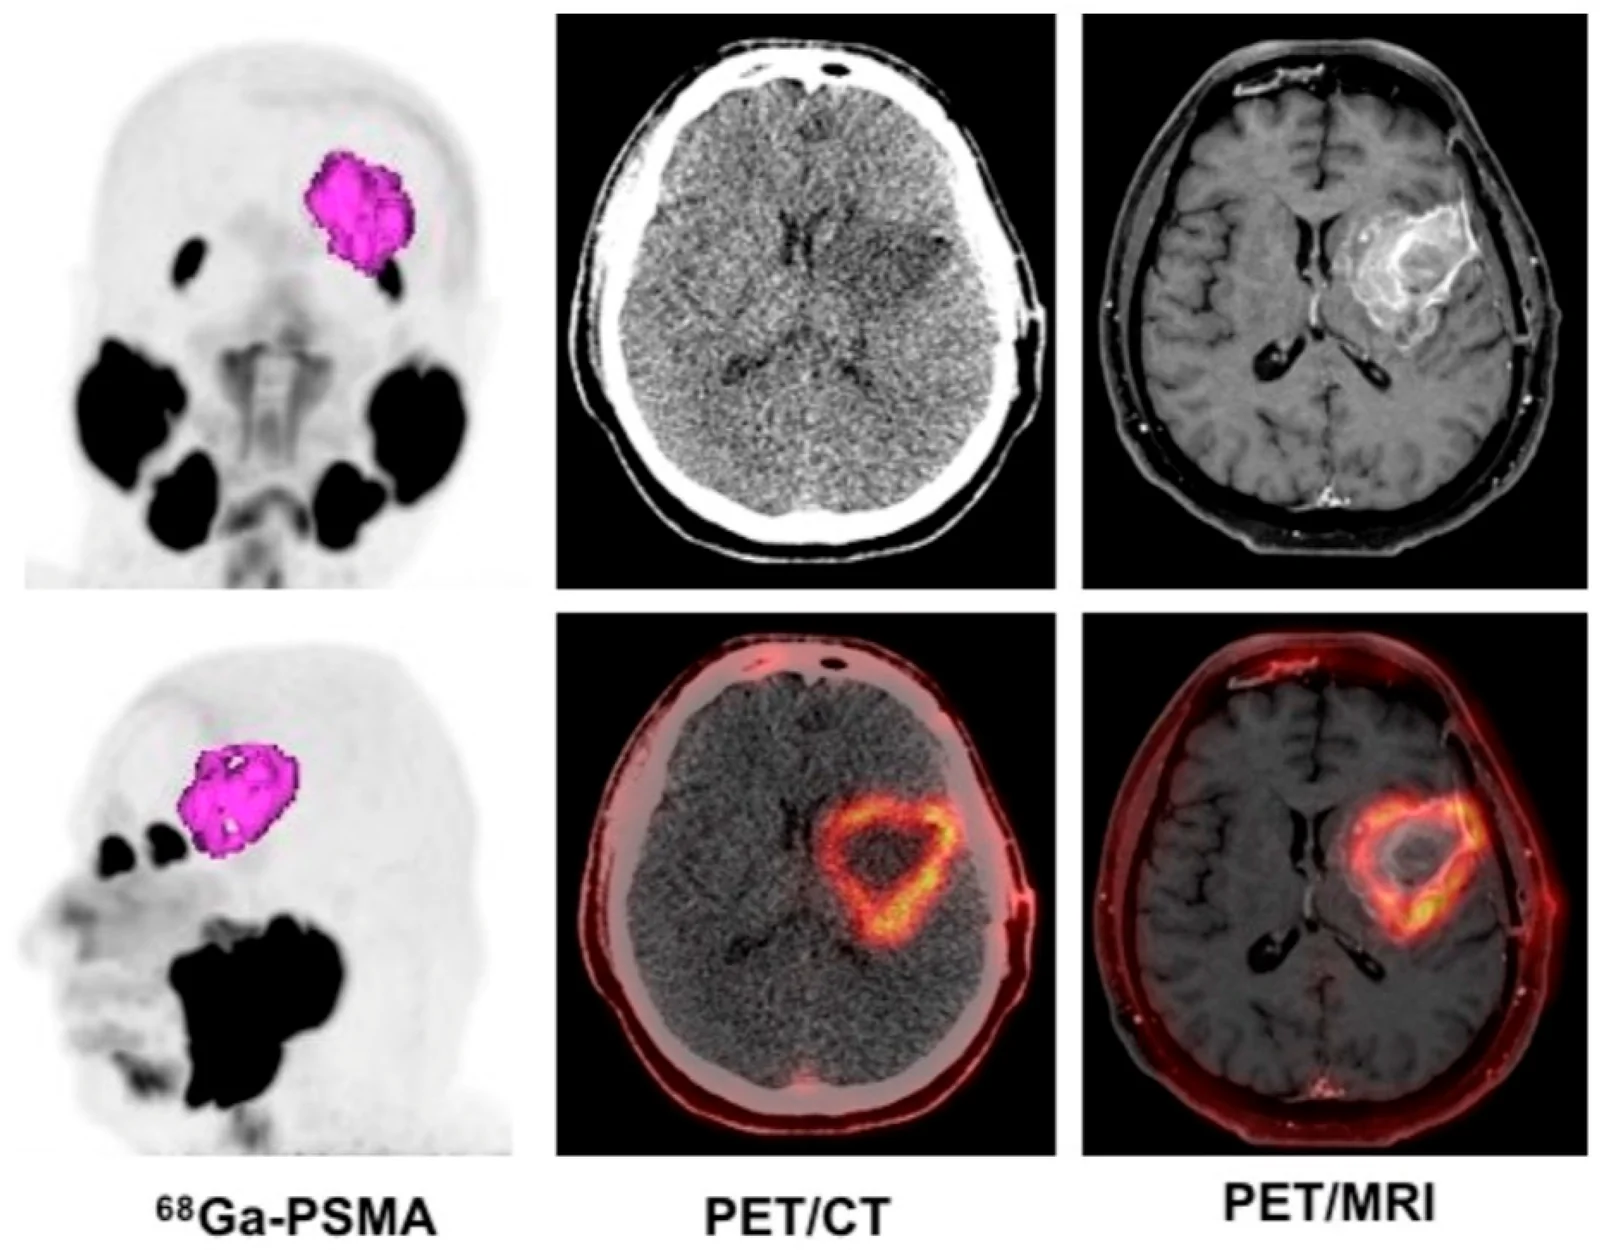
A 44-year-old male patient with glioblastoma multiforme (IDH-wildtype), treated with craniotomy: ^68^Ga-PSMA positron emission tomography (PET)/computed tomography (CT) and PET/magnetic resonance imaging (MRI) axial images show a lesion with abnormal PSMA expression in the left fronto-temporal region, with maximum standardized uptake value (SUVmax) 4.6 and metabolic tumor volume (MTV) 27.9 cm^3^ at PET/CT images. Findings are consistent with a significant active residual tumor characterized by neovascularization. Written informed consent has been obtained from the patient to publish this figure.
